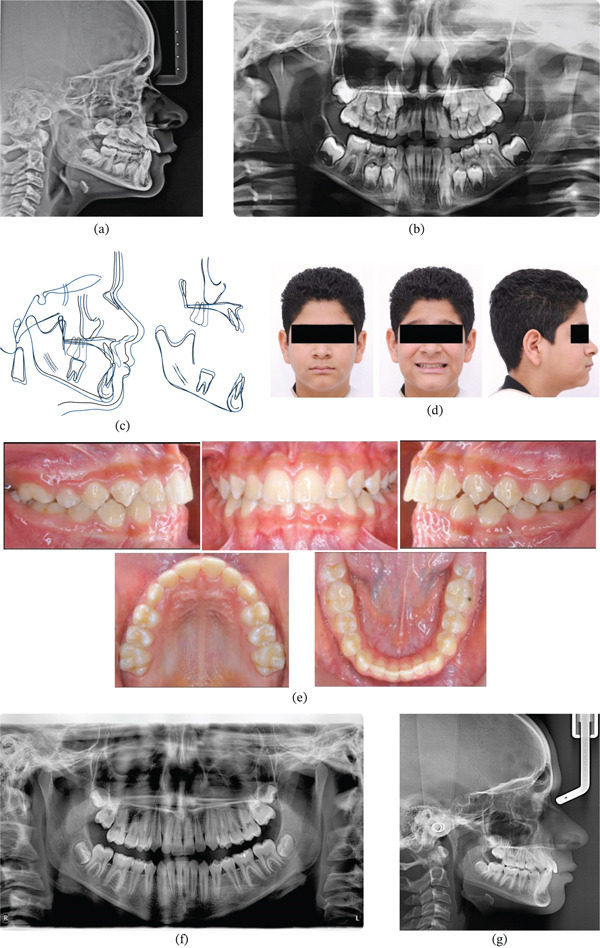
(a) Postprotraction lateral cephalogram showing improved maxillomandibular relationship. (b) Postprotraction panoramic radiograph revealing dental development progression. (c) Cephalometric superimposition comparing pre‐ and postprotraction skeletal changes. (d) Posttreatment facial photographs demonstrating final esthetic outcomes. (e) Posttreatment intraoral photographs showing Class I molar relationship and proper overjet. (f) Final lateral cephalometric radiograph confirming stable skeletal relationships. (g) Final panoramic radiograph showing complete eruption of permanent dentition.
